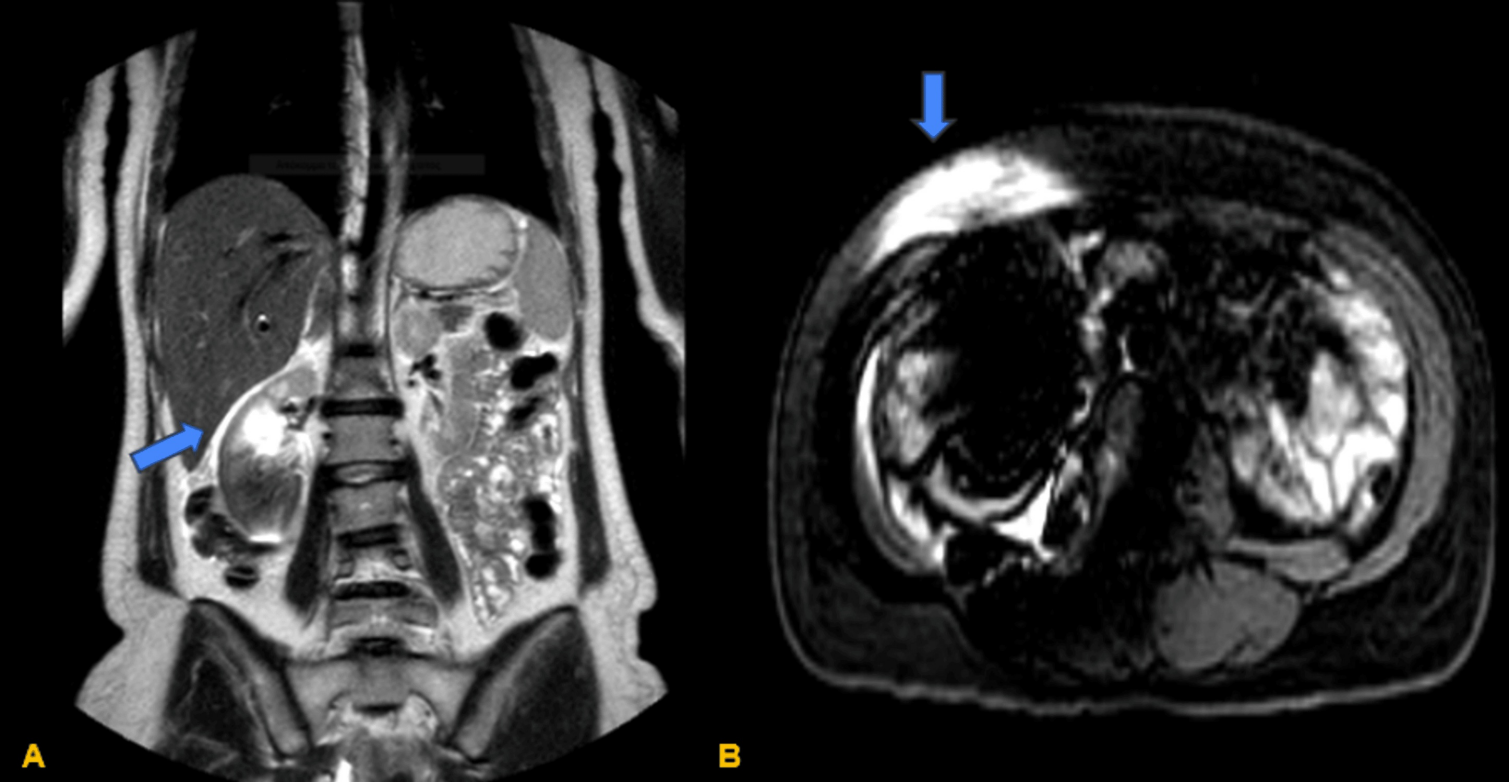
MRI scan of the abdomen. (A) Coronal view of the abdomen showing an artifact (blue arrow) in the right abdomen on the T2 sequence. (B) Transverse view of the artifact (blue arrow) caused by a metallic object discovered during the procedure on the T1 sequence
